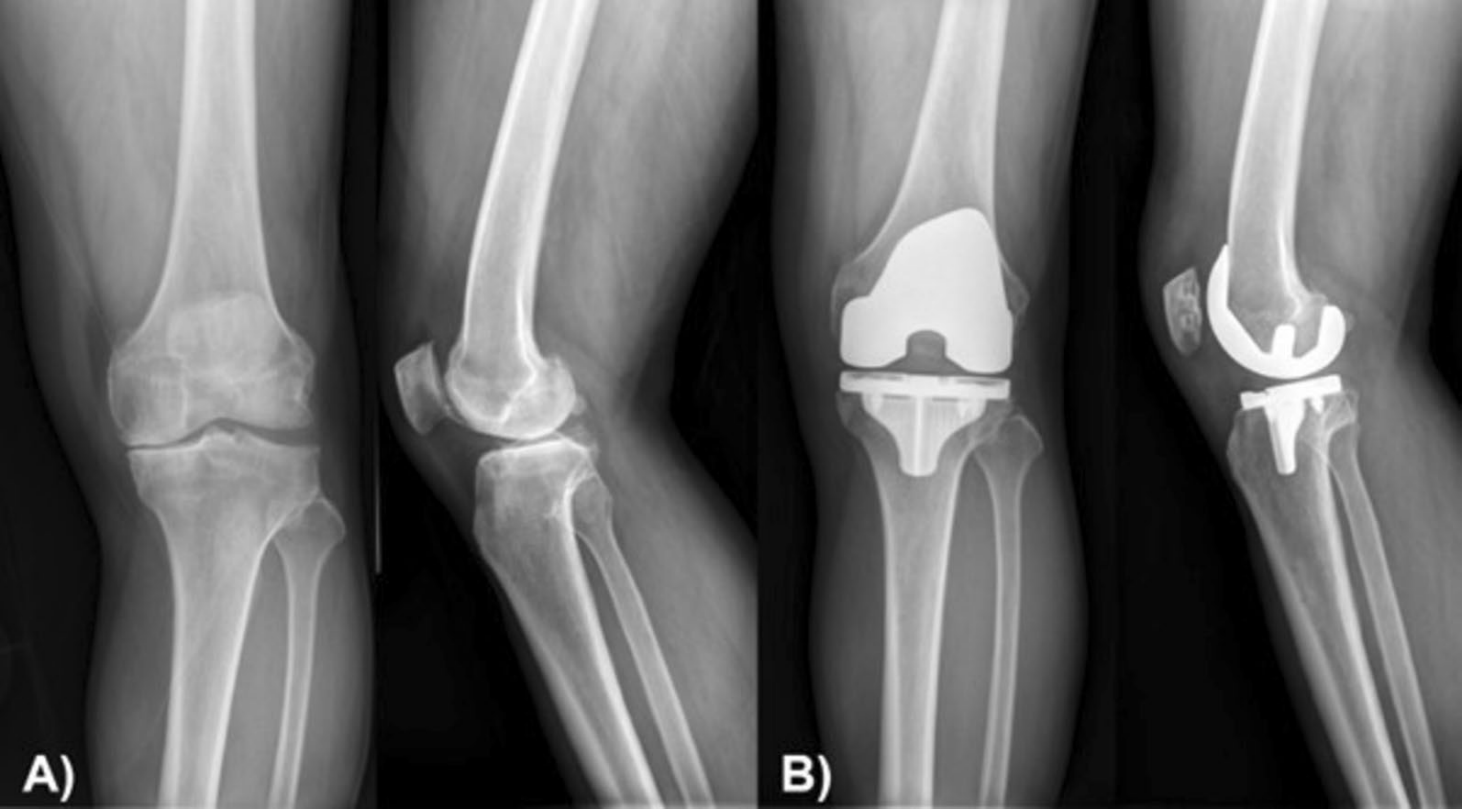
Radiographic images of a 74-year-old male patient with osteopenia and obesity (body mass index [BMI]: 30.33 kg/m^2^) undergoing left cementless total knee arthroplasty. A Preoperative X-ray of the left knee. B Postoperative X-ray of the left knee
